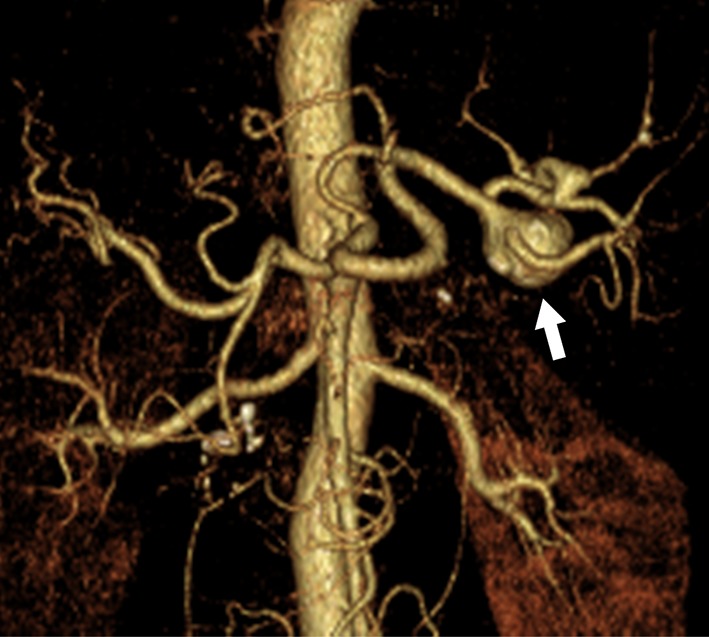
Splenic artery aneurysm in a liver transplant recipient. Three-dimensional volume rendered CT angiography shows a saccular aneurysm in the distal splenic artery aneurysm (25 mm) in a liver transplant recipient (arrow). Splenectomy was performed at the time of liver transplantation
